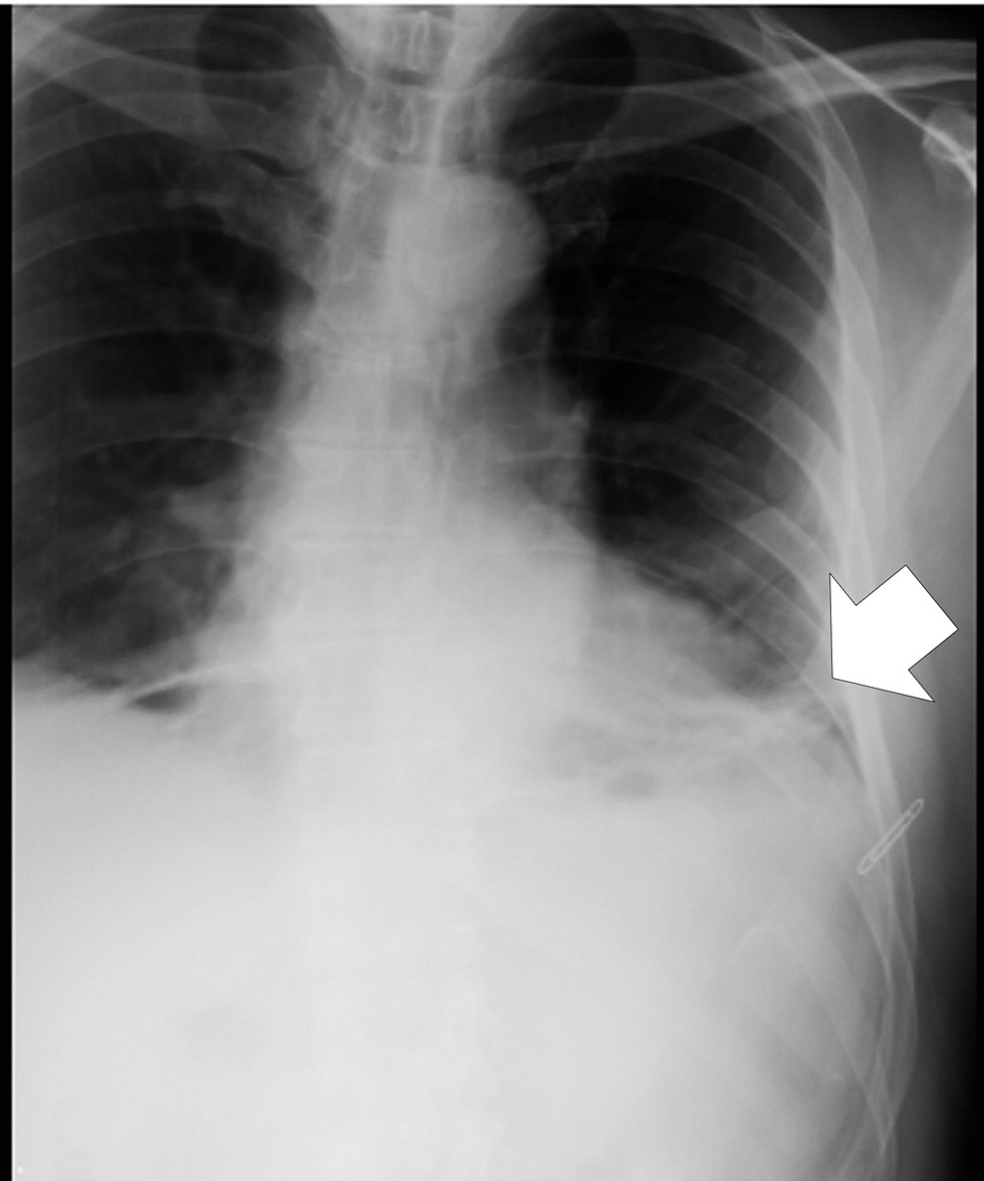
Anteroposterior chest X-ray image showing the persistence of trivial left pleural effusion performed at a family doctor (four days after the accident).
